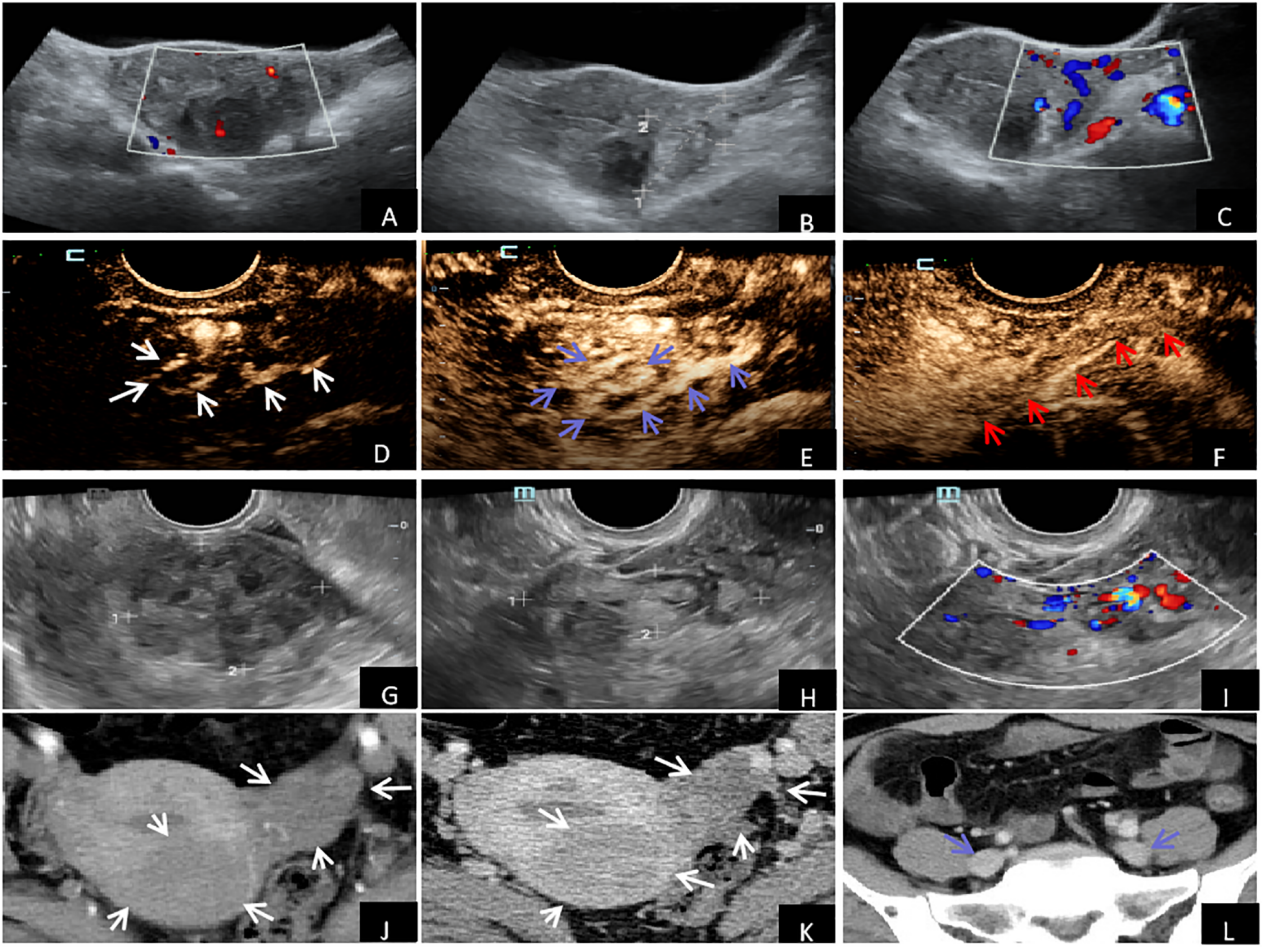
Imaging examination: (A–C) Conventional ultrasound examination showed uterine fibroids in the posterior wall, enhanced gyrus mass in the left adnexal area, and tortuous flow signals detected by CDFI. (D–F) CEUS showed synchronous and rapid hyperenhancement in the early stage of the arterial mass with enhanced echo in the left adnexal area (white arrow). During the peak stage, the interior enhancement exhibited irregularities resembling the shape of an earthworm (blue arrow). During the venous phase, the lesion exhibited reduced enhancement (hypoenhancement), whereas the outside vessel wall displayed uniform and intensified enhancement (hyperenhancement) (red arrow). (G–I) Vaginal ultrasound showed leiomyoma in the posterior wall of the uterus, with cord-like isoecho in the left adnexal area and tortuosity of blood flow signals detected by CDFI. Contrast-enhanced CT of the chest and abdomen of (J–L) showed uterine enlargement and leiomyomas in the uterus's posterior and left lateral walls (white arrows). There were no anomalies observed in the iliac vein (blue arrow).
